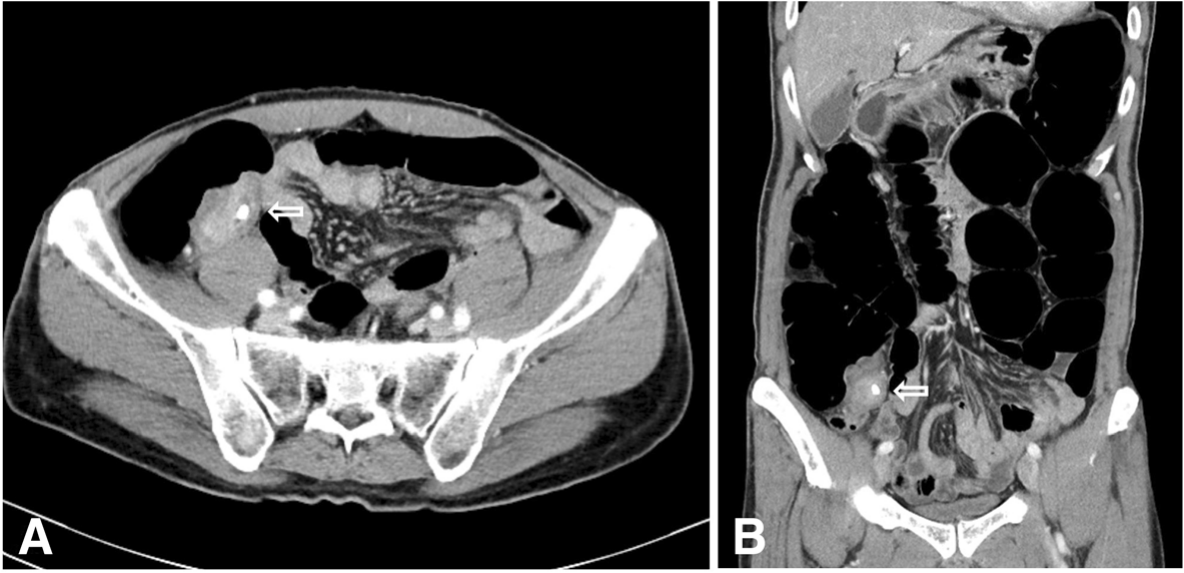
CT abdomen & pelvis images. The images show a 5 × 2.5 × 4 cm mass-like lesion in the cecum. Transverse view (a). Coronal view (b)
